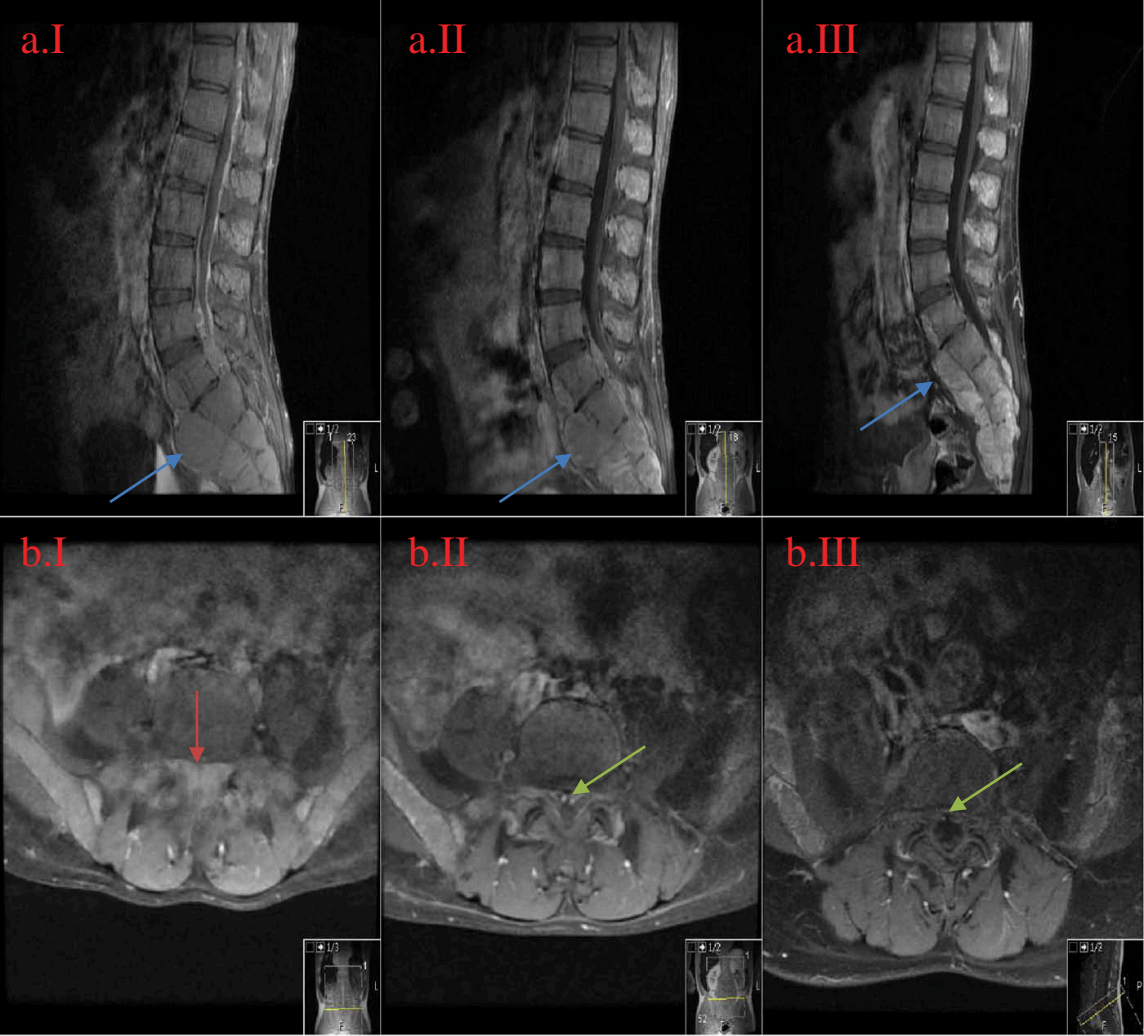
MRI of the lumbar spine in sagittal and axial views at the levels ofL5-S1. A = sagittal view; B = axial view; I = initial presentation; II = day 14th after diagnosis; III = week 11th after diagnosis. The images show regression of the central spinal canal stenosis: from near-complete-obliteration on the initial image (B.II.1 red arrow) to the subsequent re-emergence of the spinal canal (B.II.2 and B.II.3 green arrow) with medical treatment. Also noted is the decrease in size of the bony masses and is the most prominent in the sacral region (II.A images blue arrow).
